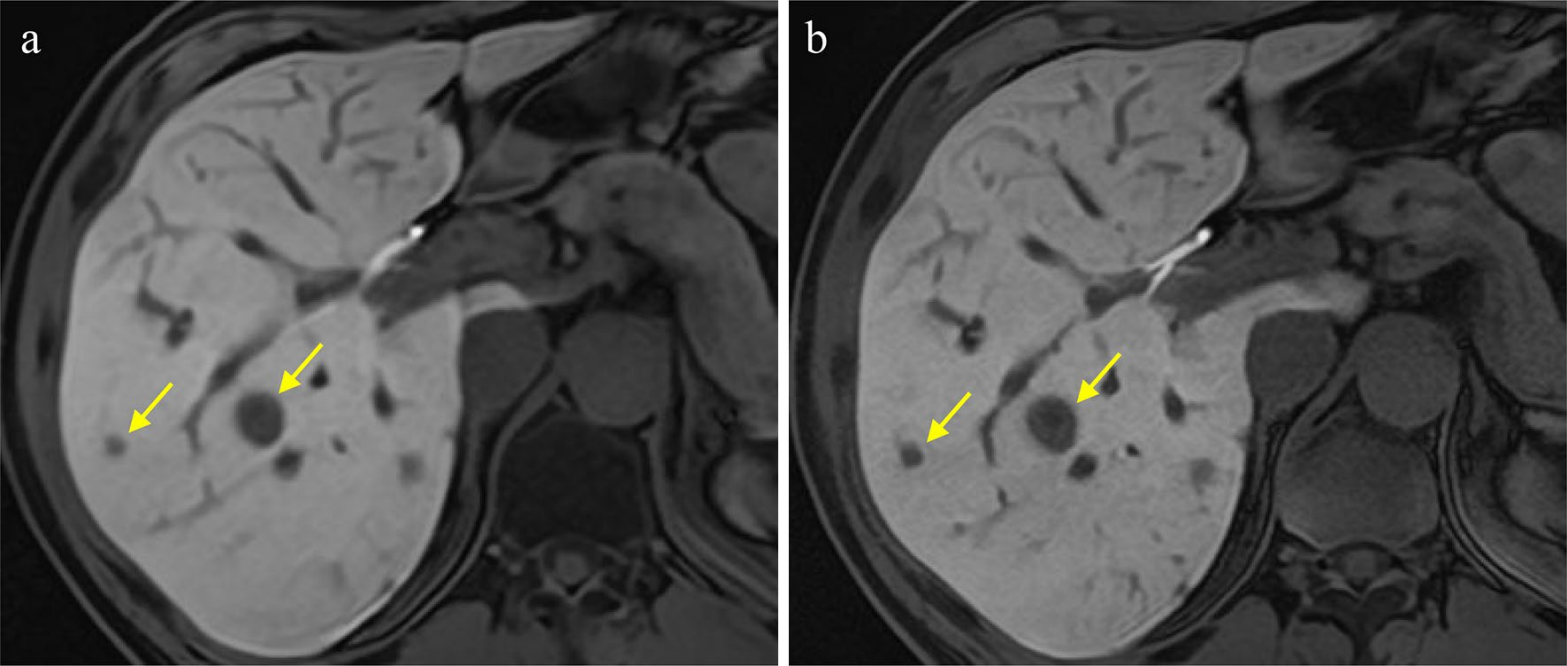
MR images in a 54-year-old man with multiple liver metastases from pancreatic cancer. a HBP image with CS using AiCE. b High-precision HBP image with modified Fast 3D mode wheel and PIQE. Conspicuity of intrahepatic vascular structures, bile duct and metastatic tumors (arrows) was better in high-precision HBP image with modified Fast 3D mode wheel and PIQE (b), compared with HBP image with CS using AiCE (a)
